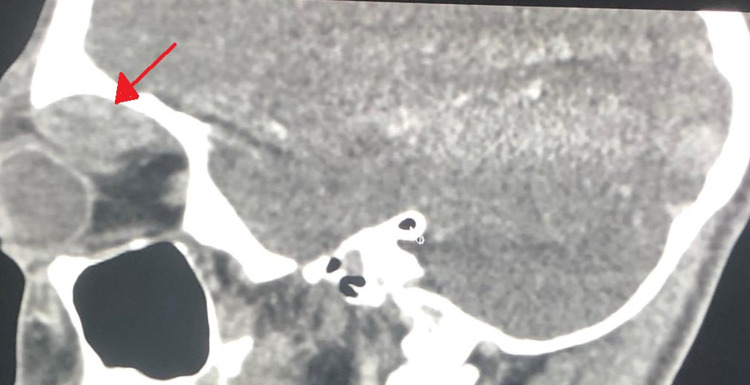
Sagittal computed tomography scan showing left superior extraconal cystic mass (red arrow) compressing the globe causing dystopia and proptosis.
